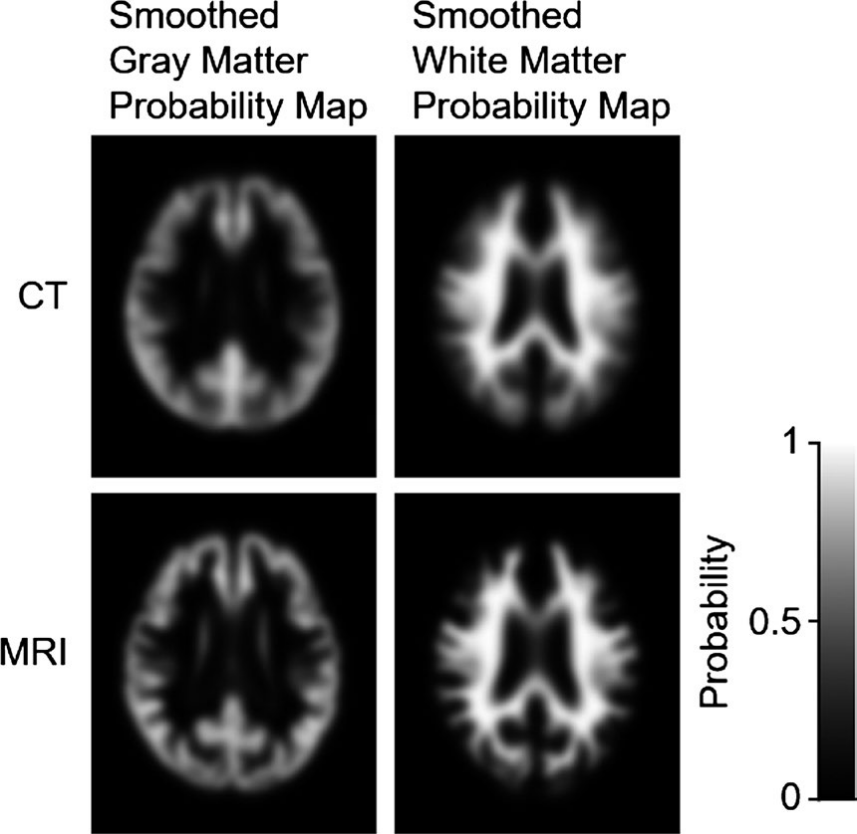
Examples of the smoothed probability maps obtained from the CT and MR image of a single subject. Top left: GM from CT; top right: WM from CT; bottom left: GM from MRI; and bottom right: WM from MRI
